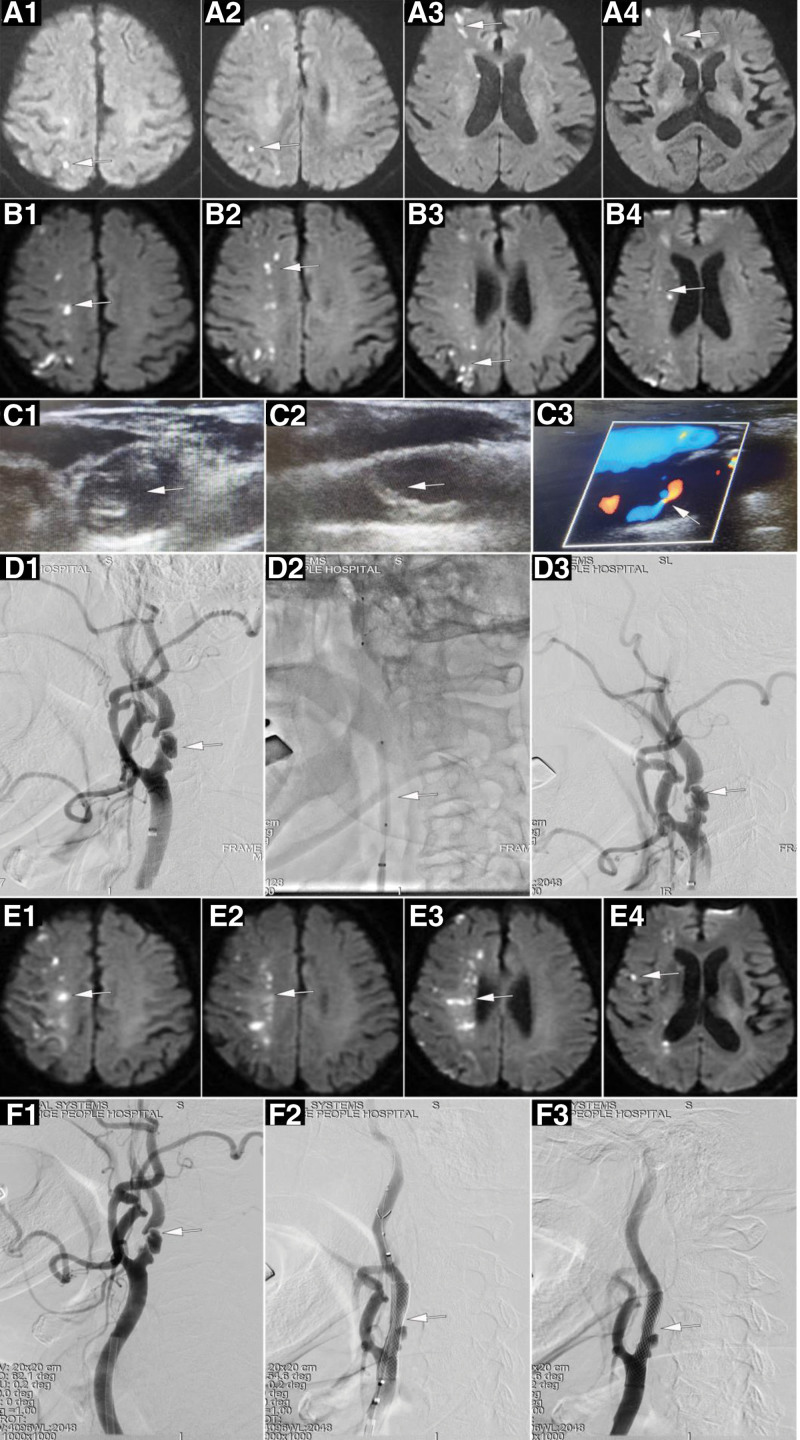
A 83-year-old man experienced a sudden onset of left-sided limb weakness of unknown origin. Diffusion-weighted image (DWI) showed Multiple spot-like infarcts in the right cerebral hemisphere (A1–A4). A follow-up DWI indicated more infarcts than before (B1–B4). Color Doppler ultrasound revealed severe stenosis of the right internal carotid artery (C1–C3). Left internal carotid artery was dilated by balloon (D1–D3). The patient experienced a recurrence of left-sided limb weakness, subsequent head MRI revealed a further enlargement of the infarction area (E1–E4). CAS was performed using WALLSTENT endoprosthesis (F1–F3).
